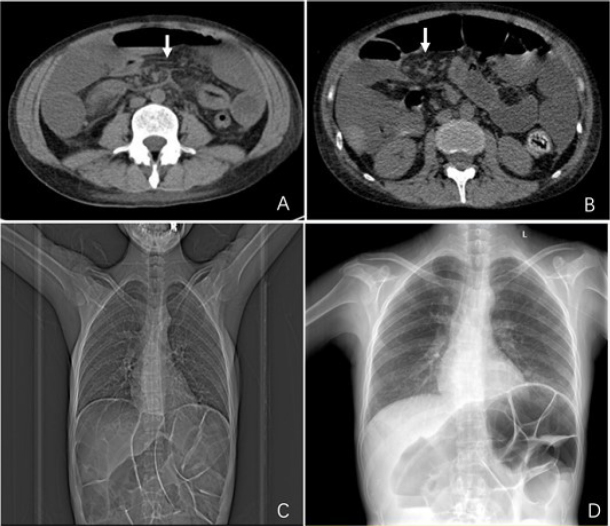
Computed tomography scan of the abdominal. The whirl sign (arrow) under abdominal X-ray (A and B) before treatment (C) and six months after treatment (D).
